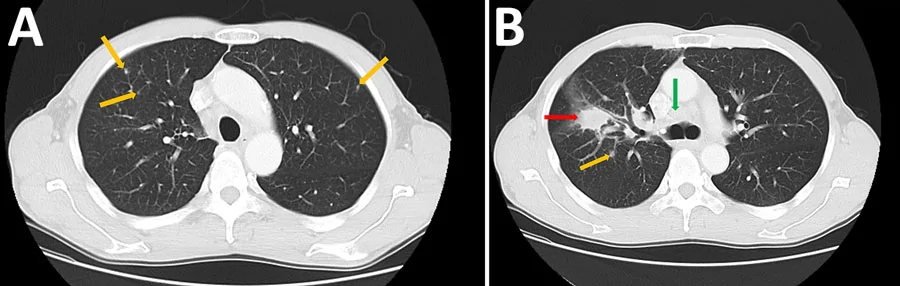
Chest computed tomography findings in a maritime container yard worker with pulmonary coccidioidomycosis, Taiwan, 2025. A) Axial lung-window images reveal multiple bilateral pulmonary nodules (orange arrows). B) A 3.0 × 2.0 cm solid mass is visible in the right upper lobe (red arrow), and right hilar lymph nodes are enlarged (green arrow).
